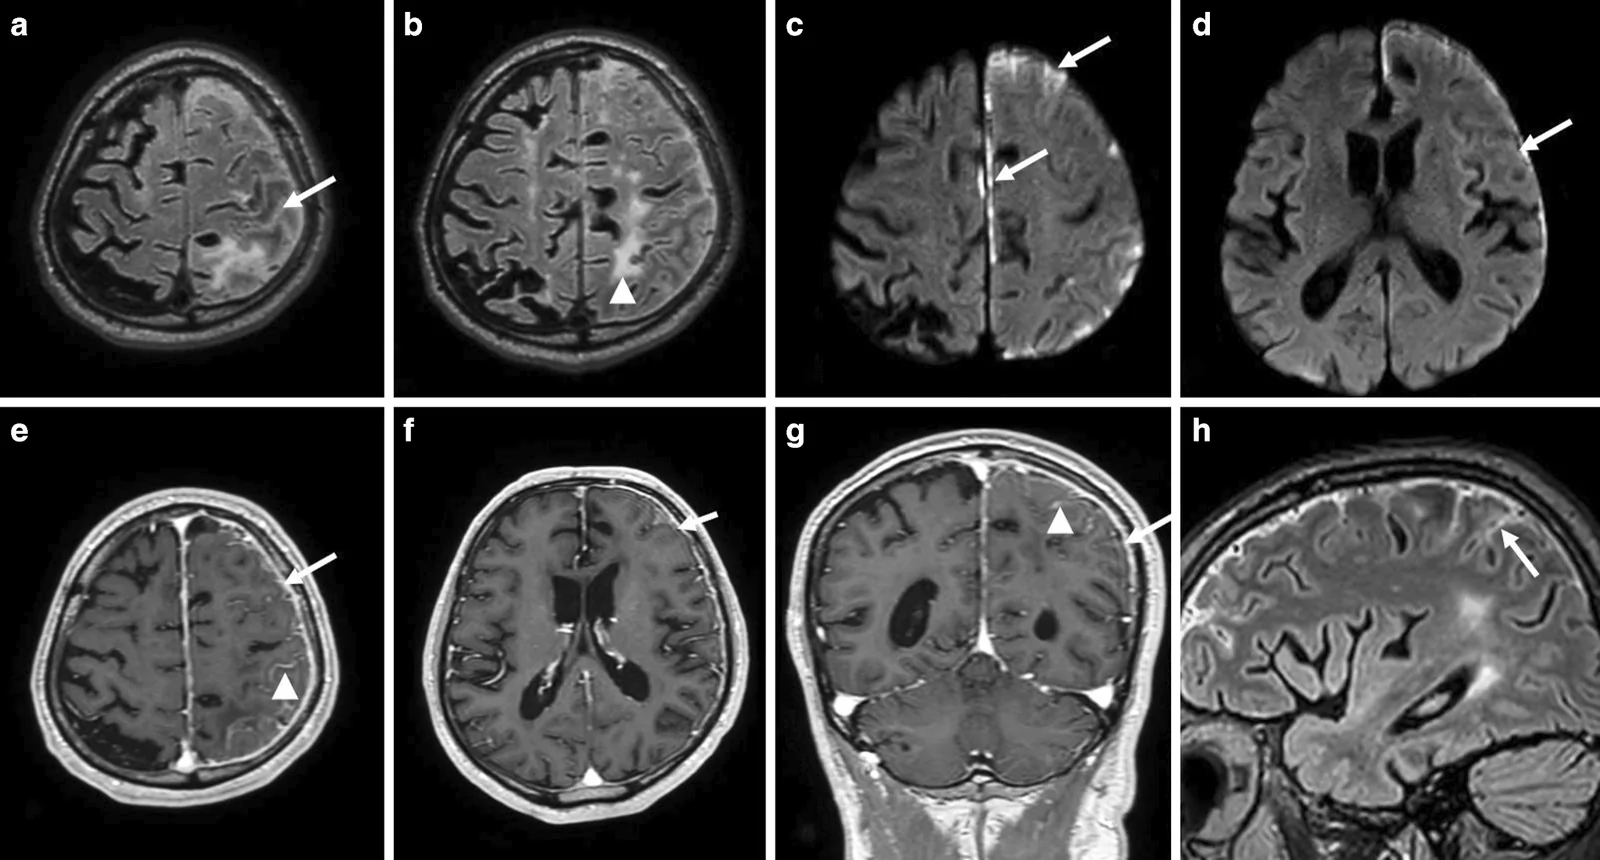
IgG 4 related biopsy proven hypertrophic pachymeningitis in a 61 year old man with slight right-sided hemiparesis and first epileptic seizure. a–h sulcal effusion (a, h): arrow; fluid attenuated inversion recovery (FLAIR) and adjacent cortical and subcortical hyperintense signal changes (b arrowhead); diffusion restriction (c, d arrow; diffusion weighted imaging (DWI), b = 1000 s/mm^2^) and distinct contrast enhancement (CE) of the thickened pachymeninx (e–g arrow). Note also slight CE of leptomeningeal structures (e, g arrowhead)
